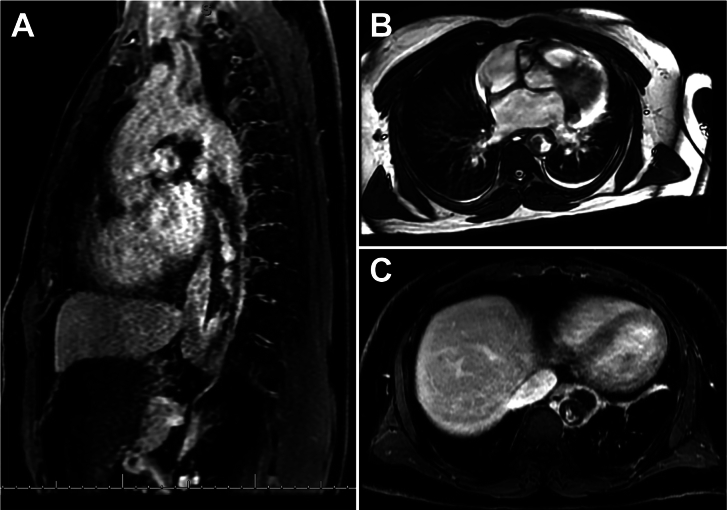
(A) Magnetic resonance angiogram (MRA) in sagittal plane after contrast enhancement. (B) Axial MRA, T1-weighted image before contrast enhancement. (C) Axial MRA, T1-weighted image after contrast enhancement.
